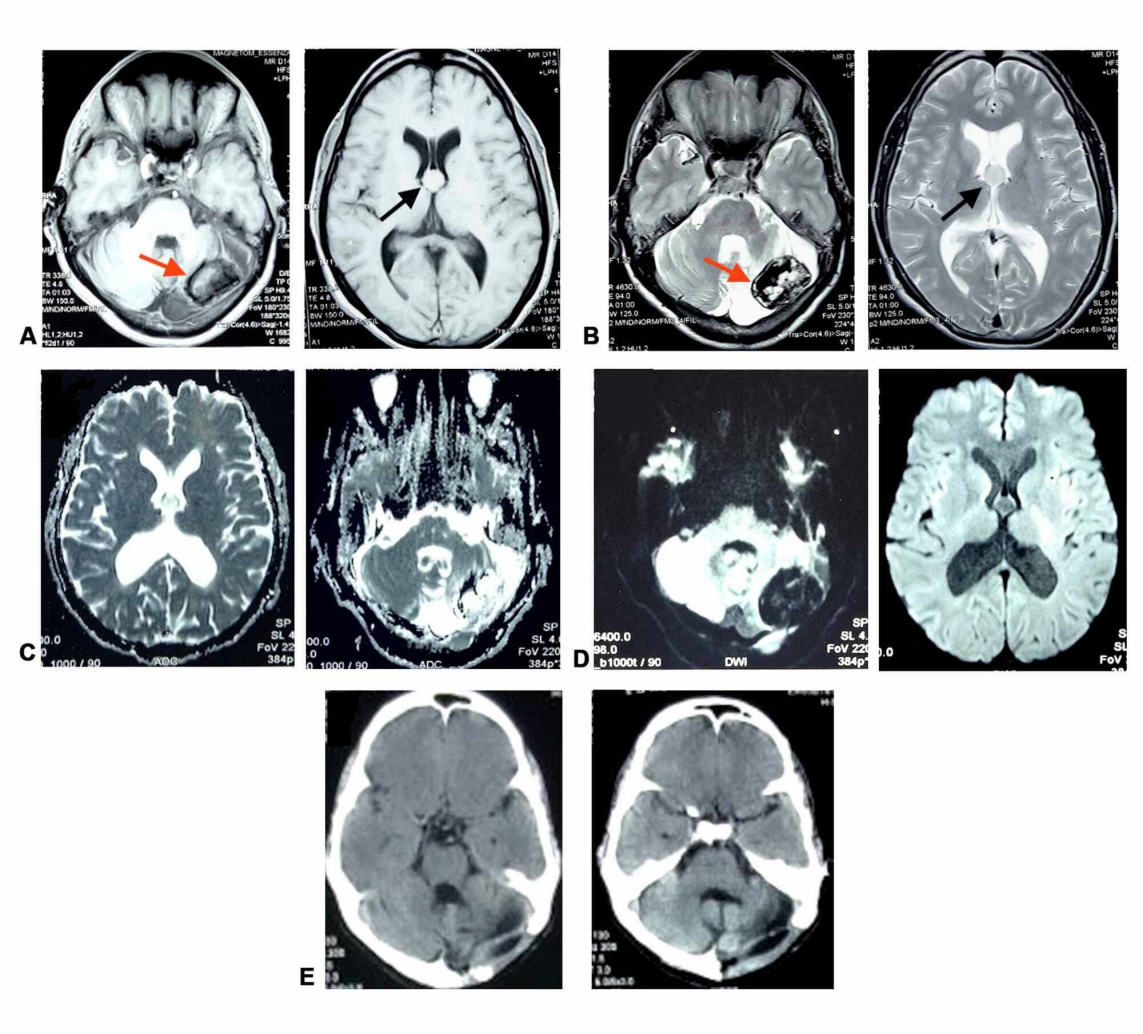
Well-defined extra-axial heterogeneous non-enhancing lesion seen in left cerebellar convexity with peripheral T1 and T2 hypointense rim and internal components showing mixed T2 hypo- and hyperintensity (red arrow). Left cerebellar atrophy is also seen. Another well-defined mildly T1 hyperintense and T2 isointense lesion is seen at the foramen of Monro, suggestive of colloid cyst (black arrow) (Figures A and B). Figures C and D demonstrate the DWI and the ADC sequences. (E) Post-operative CT scan following left sub-occipital craniectomy, showing complete excision of fibromyxoid cerebellar lesion. The hyperdense colloid cyst lesion can be seen. DWI, diffusion-weighted images; ADC, apparent diffusion coefficient; CT, computed tomography
